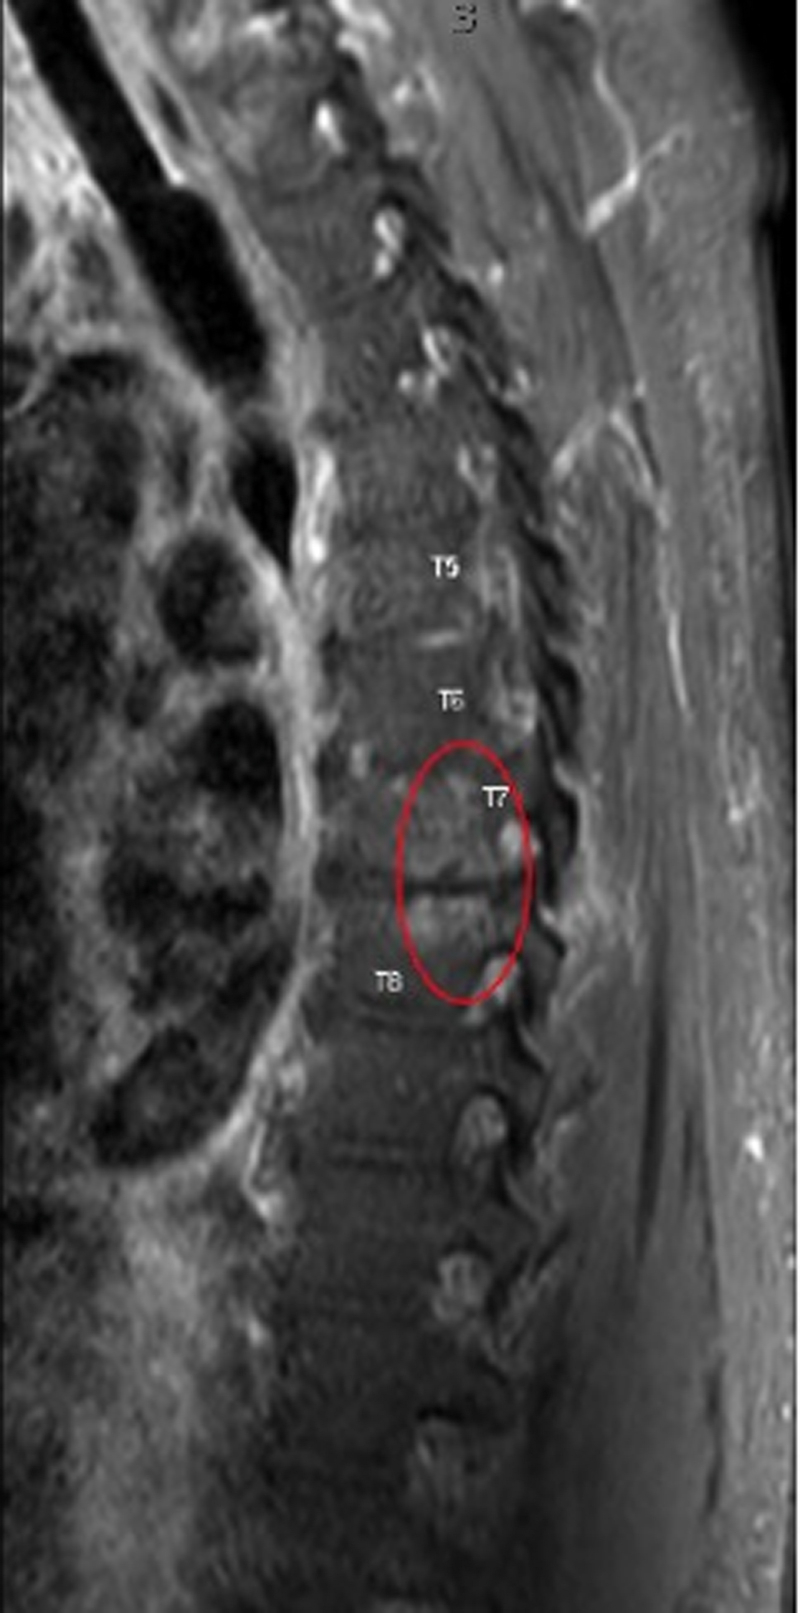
MRI of Thoracic Spine With Osteomyelitis Sagittal postcontrast T1-weighted MRI of the thoracic spine demonstrates enhancement in the T5-T8 vertebral bodies (red ellipse) consistent with osteomyelitis. MRI = magnetic resonance imaging.
